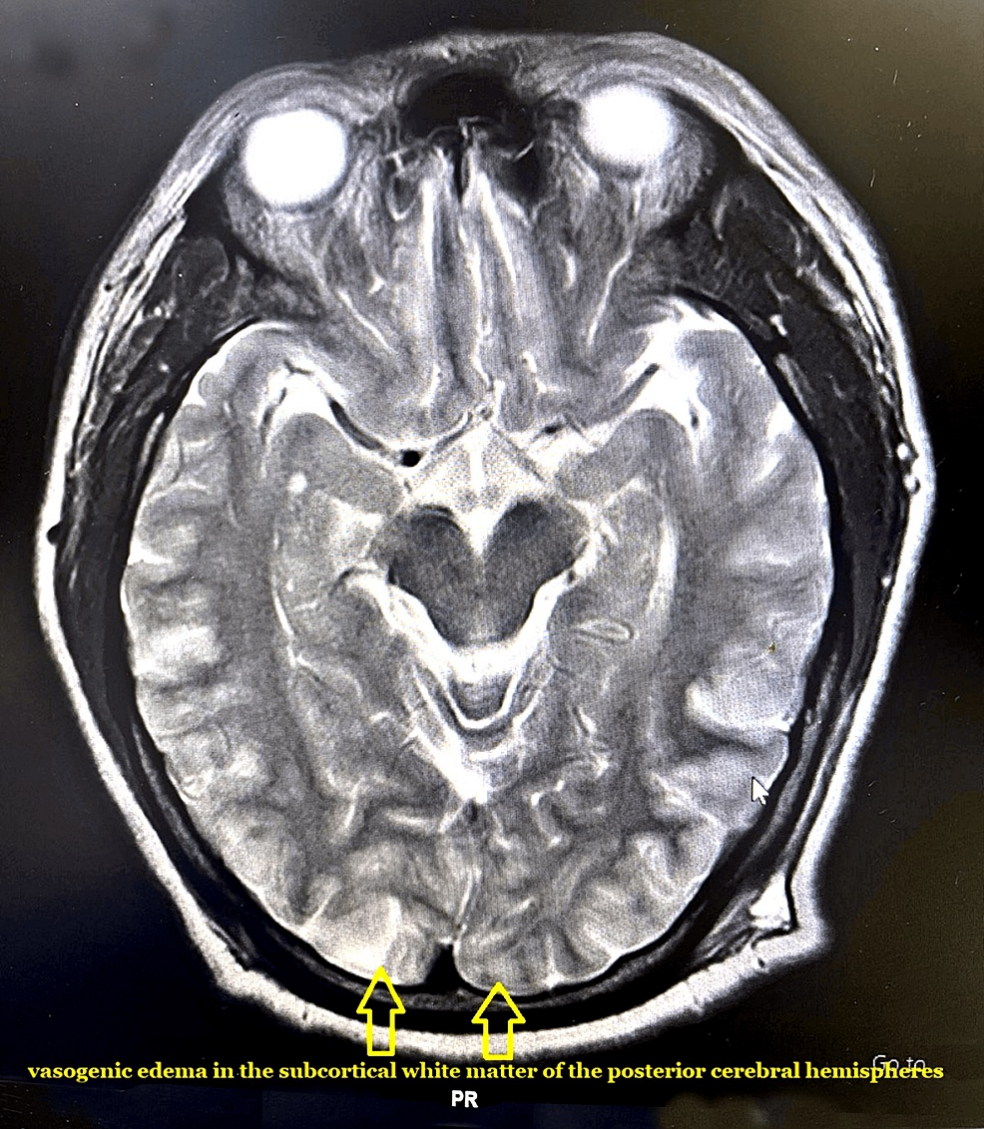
CT image suggestive of posterior reversible encephalopathy syndrome
